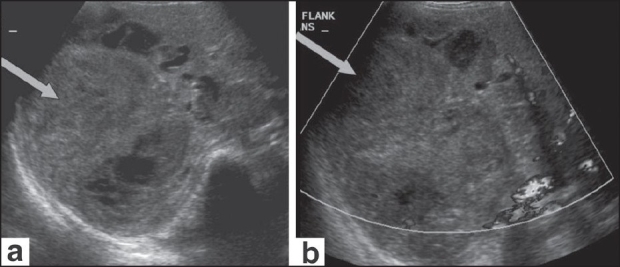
USG of the abdomen (a) reveals a large, complex, solid-cystic mass (arrow) within the right lobe of the liver, appearing predominantly solid in appearance. The color Doppler image (b) shows absence of increased vascularity in the mass lesion (arrows)
